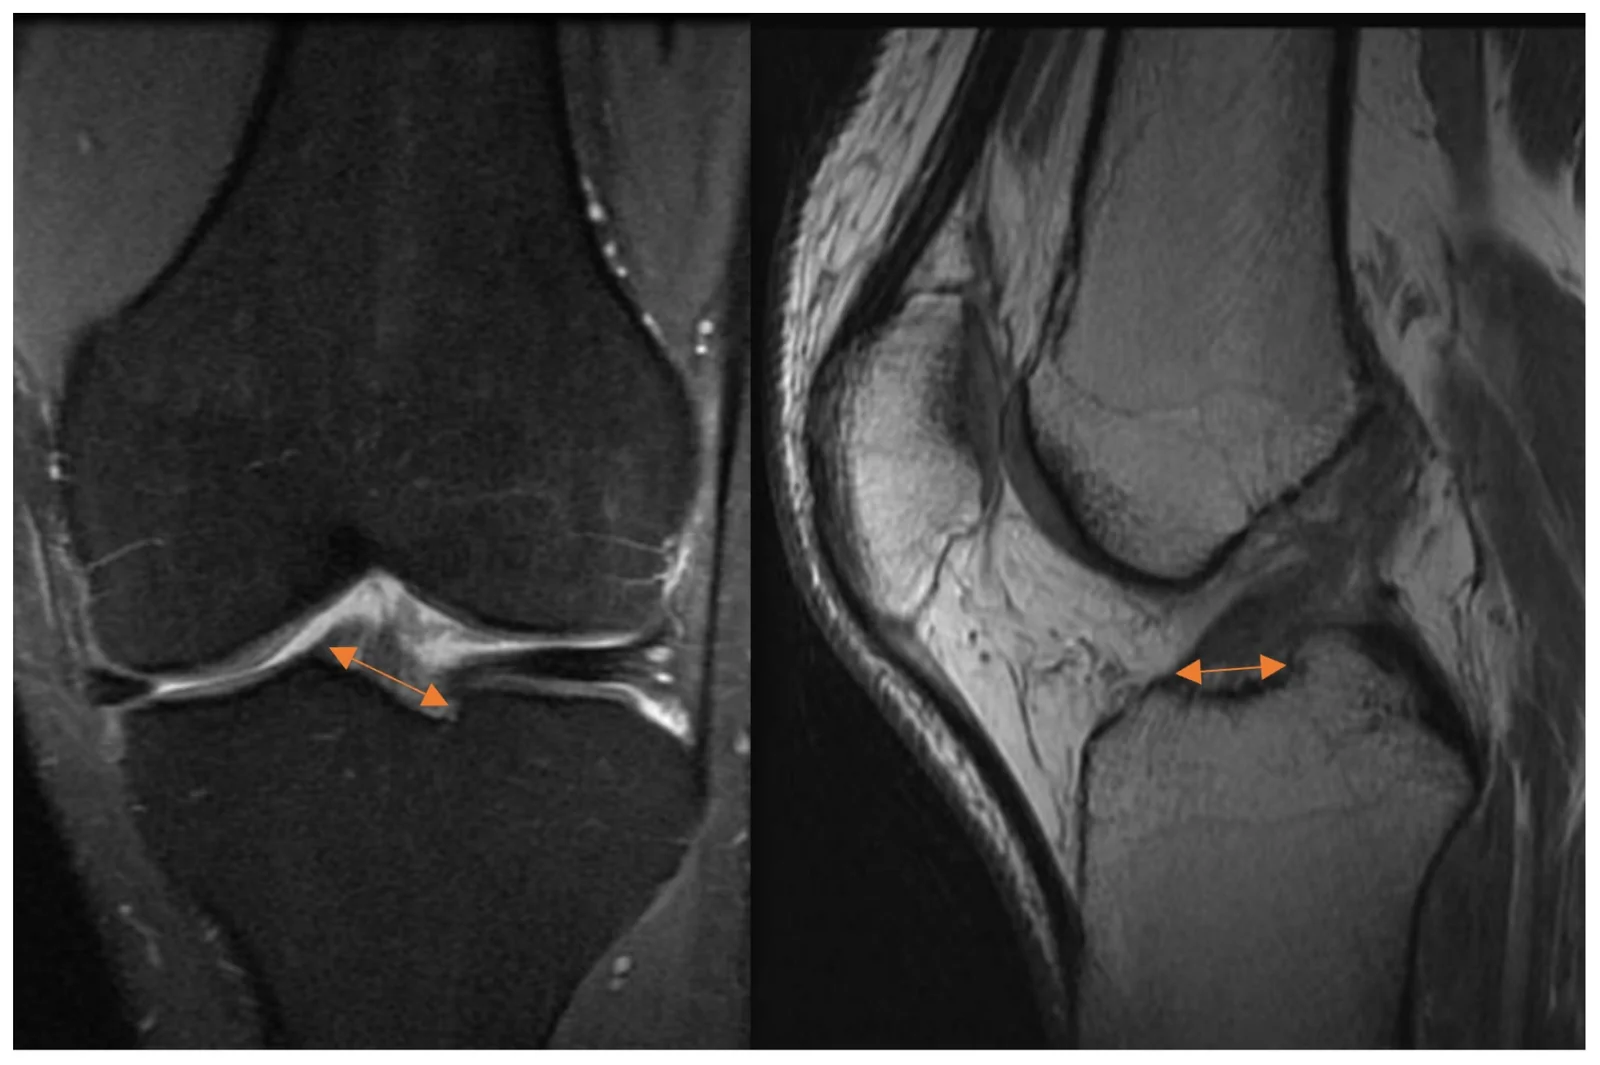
Estimation of native ACL footprint anteroposterior and mediolateral lengths based on coronal and sagittal cuts on pre-operative magnetic resonance imaging (MRI) scans.
